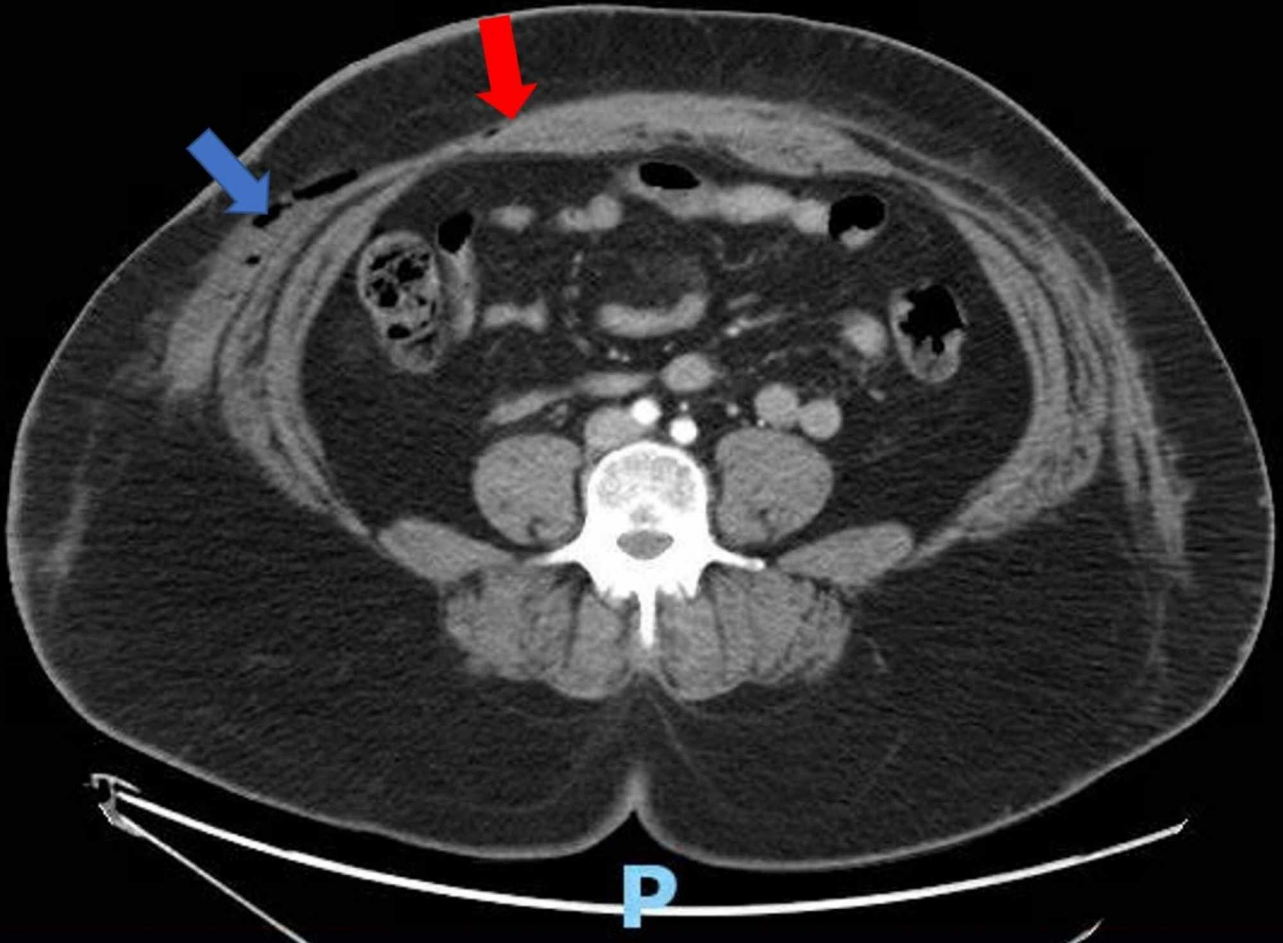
Computed tomography (CT) scan: transverse view of the abdominal cavity. Mixed attenuated fluid collection noted in the right anterolateral abdominal wall (blue arrow), with connection to the midline fluid collection (red arrow). The letter P denotes the posterior orientation of the patient.
